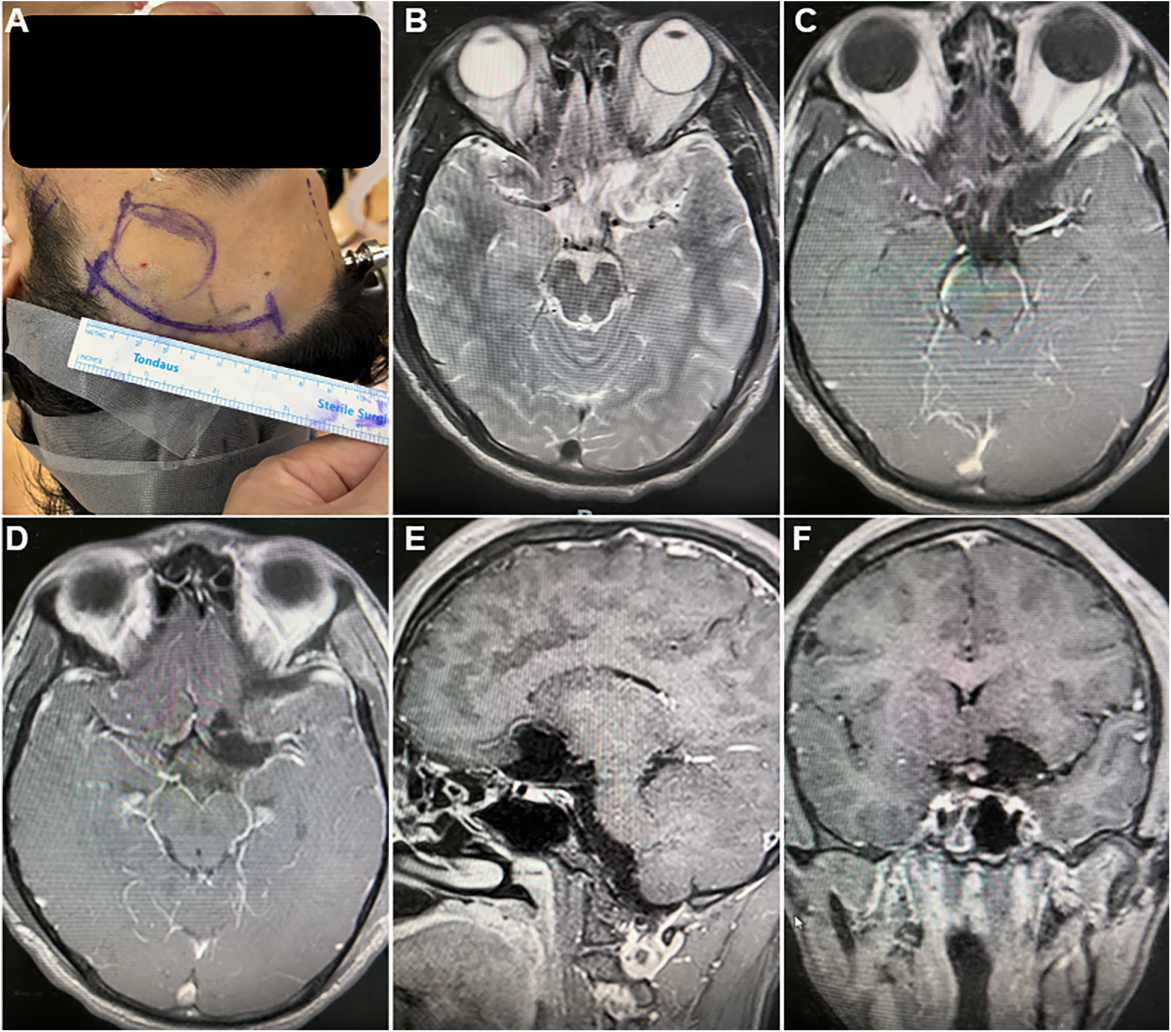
Surgical incision and postoperative magnetic resonance image. (A) the curvilinear surgical incision within the left frontotemporal hairline. (B–F) postoperative magnetic resonance image revealed complete resection of the tumor, with no residual tissue, hemorrhage, or fluid accumulation: (B) T2-weighted image (axial section); (C–F) Contrast-enhanced T1-weighted image (C,D) axial section; (E) sagittal section; (F) coronal section.
